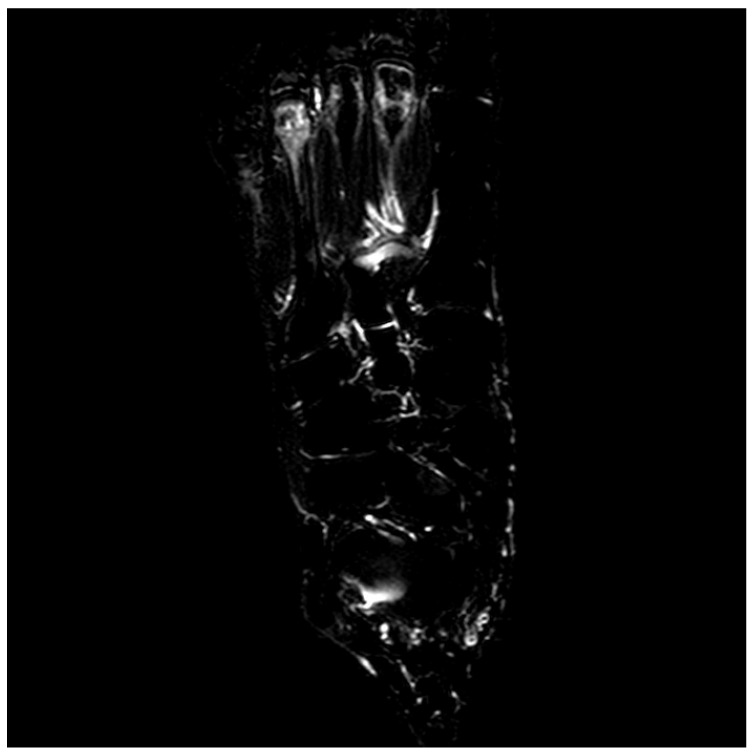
Fat-saturated T2-weighted MRI showing edema-like marrow hyperintensity at the fourth metatarsal head in a 40-year-old patient with persistent atraumatic foot pain unresponsive to NSAID therapy.
